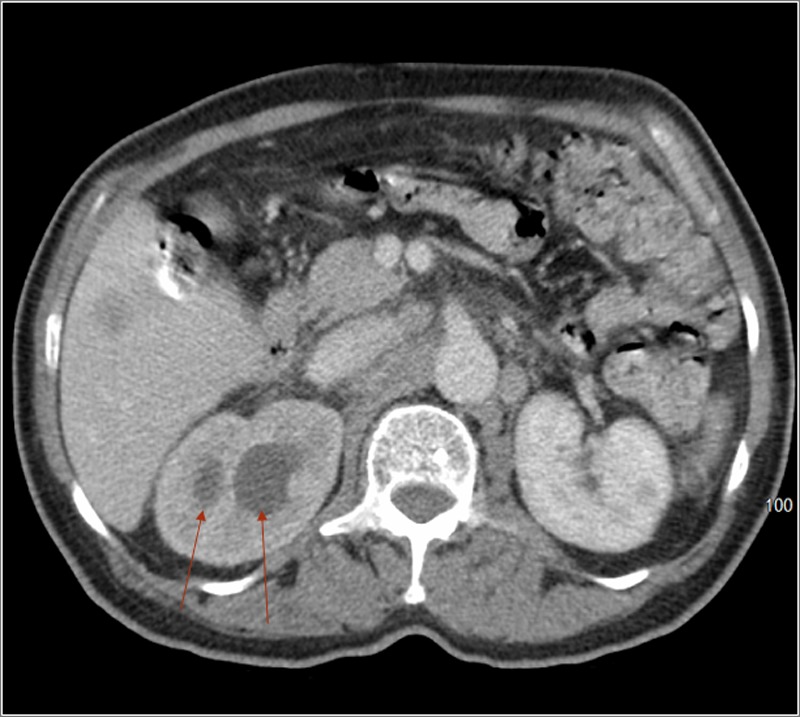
Axial view of CT scan with contrast showing delayed nephrogram and hydronephrosis of both collecting systems (see *arrows*) on the right kidney.
